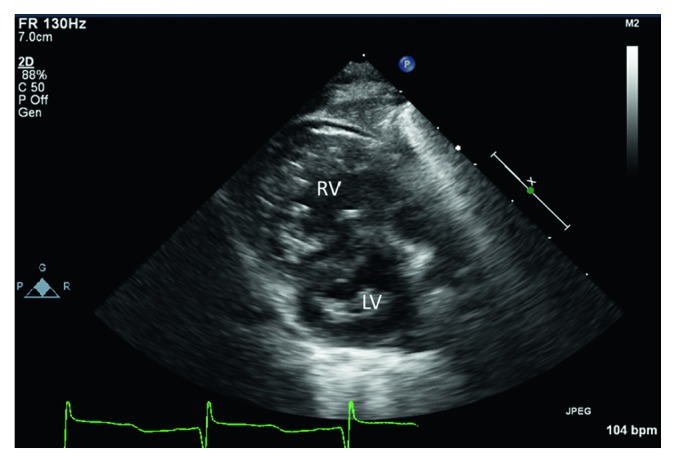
Parasternal short-axis view showing a flattening of the intraventricular septum during systole. Enlargement and hypertrophy of the right ventricle are also shown. RV, right ventricle; LV, left ventricle.
